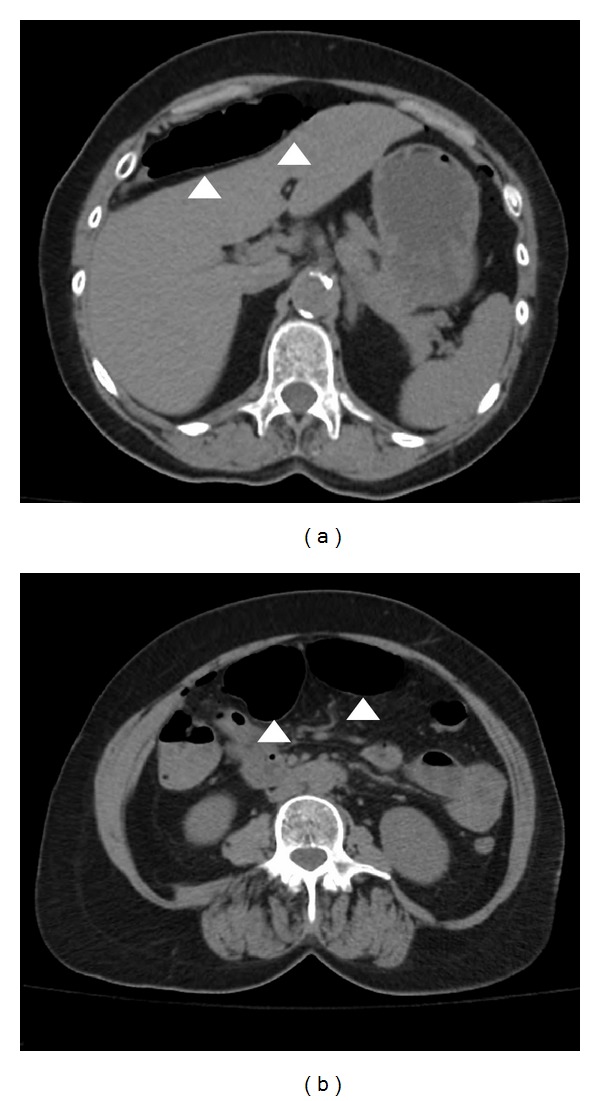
Abdominal CT scan images of a patient with colonoscopic perforation. The images show intraabdominal free air (arrowheads).
